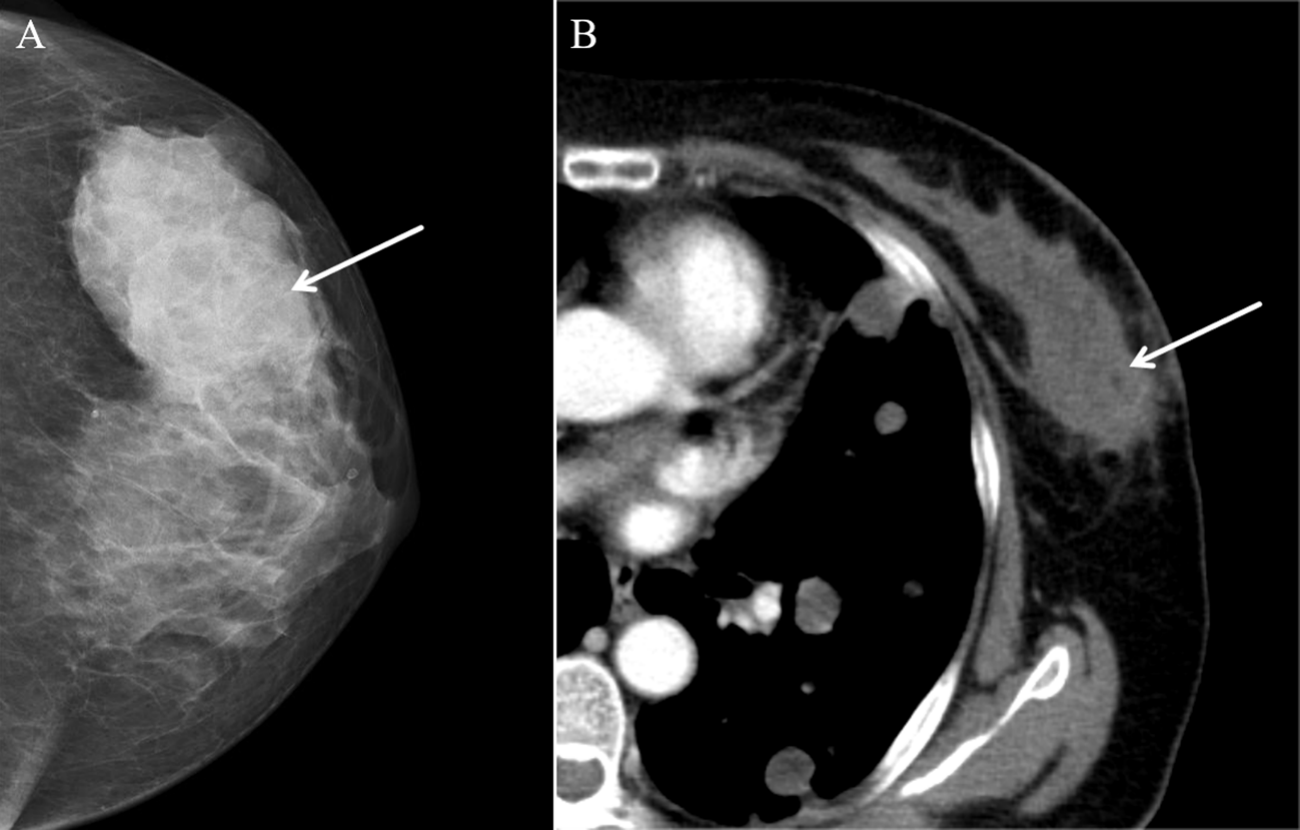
Mammography and contrast-enhanced CT (CE-CT) findings of malignant glomus tumor in the breast. (A) Axial mammography image showing a high-density lesion (arrow). (B) CE-CT revealed a poorly defined soft tissue mass (arrow).
